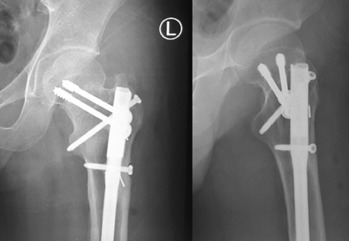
Plain radiographs of the patient after the Miss-A-Nail technique screw fixation for the femoral neck fracture. Note that the remnants of the broken 2.0mm threaded guide wire are not retrieved as they are embedded inside the femoral bone.
